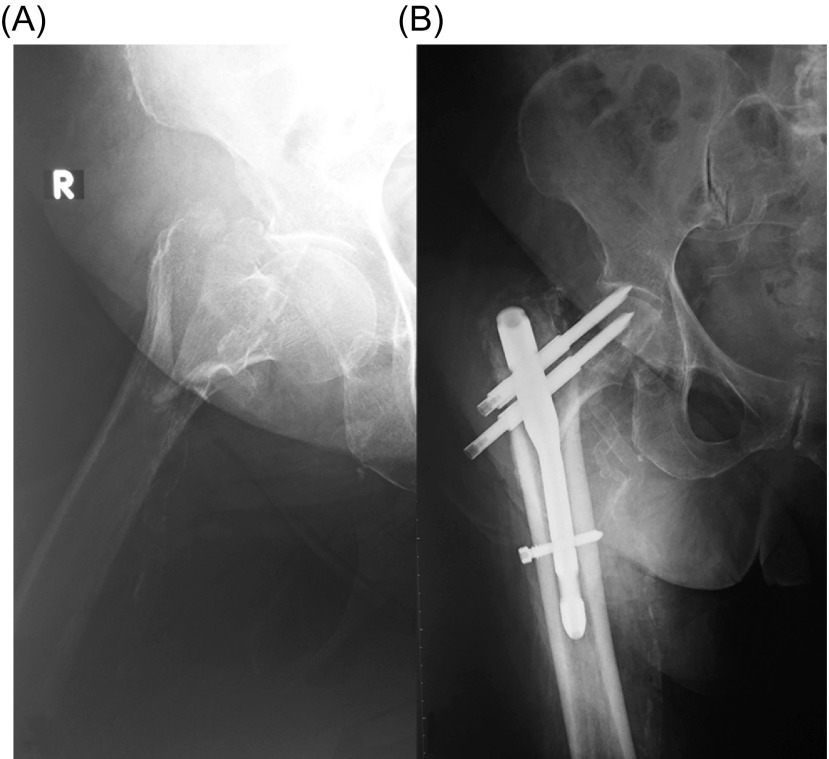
(A) Anteroposterior radiograph of the right hip of an 85-year-old woman shows an AO/OTA-31-A2 fracture. (B) Anteroposterior radiograph of the right hip eight weeks after surgical treatment shows screw cut-out and z-effect. The patient was alive with poor function and did not consent to further treatment.
